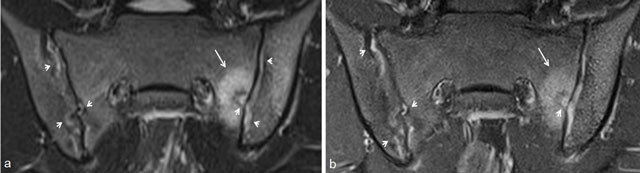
Active and structural sacroiliitis in a 17-year-old girl with JSpA: no added value of Gd. a. Semicoronal STIR image shows high signal in the joint space at both sides and in the erosions (small arrows) and BME on the left sacral side (large arrow). b. Contrast-enhanced fat-saturated T1-weighted image shows synovial enhancement in both SI joints in the erosions (small arrows) and enhancing osteitis on the left (large arrow).
